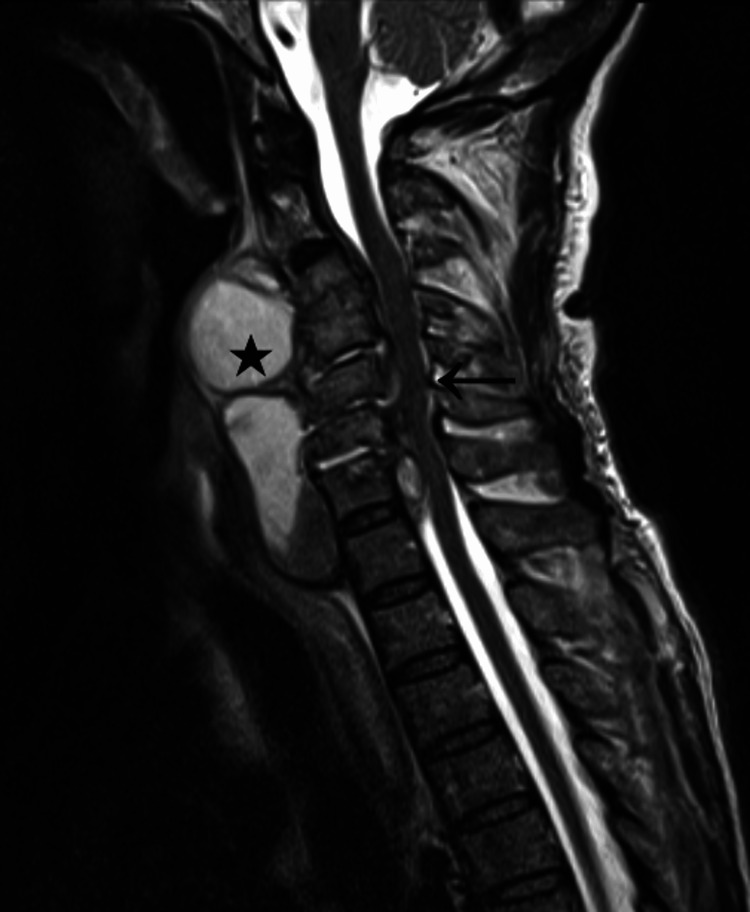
Sagittal section of the spine on MRI: the star shows the retropharyngeal abscess and the arrow shows the formation of the spinal cord.
